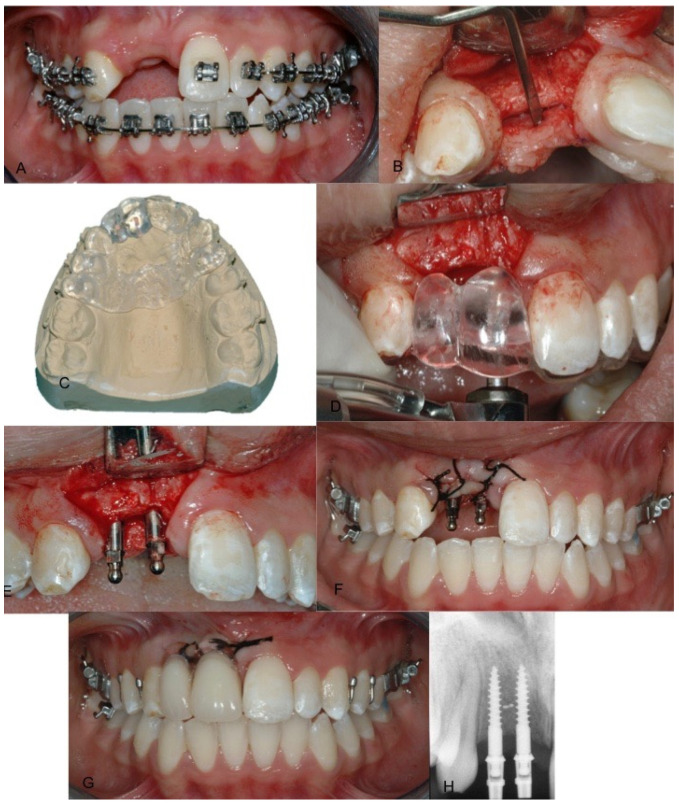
(A) Buccal clinical view after 4 months from extractions, (B) crestal bone volume, (C) surgical guide and upper model, (D) intraoral surgical guide placement, (E) paralleling during implant placement, (F) suture, (G) removable prosthesis placement and (H) immediate periapical X-ray control.
